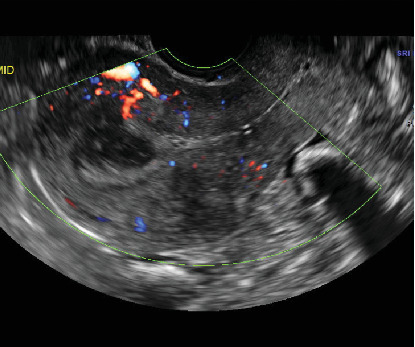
Postabortum transvaginal ultrasound showing resolving retained products of conception and low vascular flow inconsistent with AVM.
